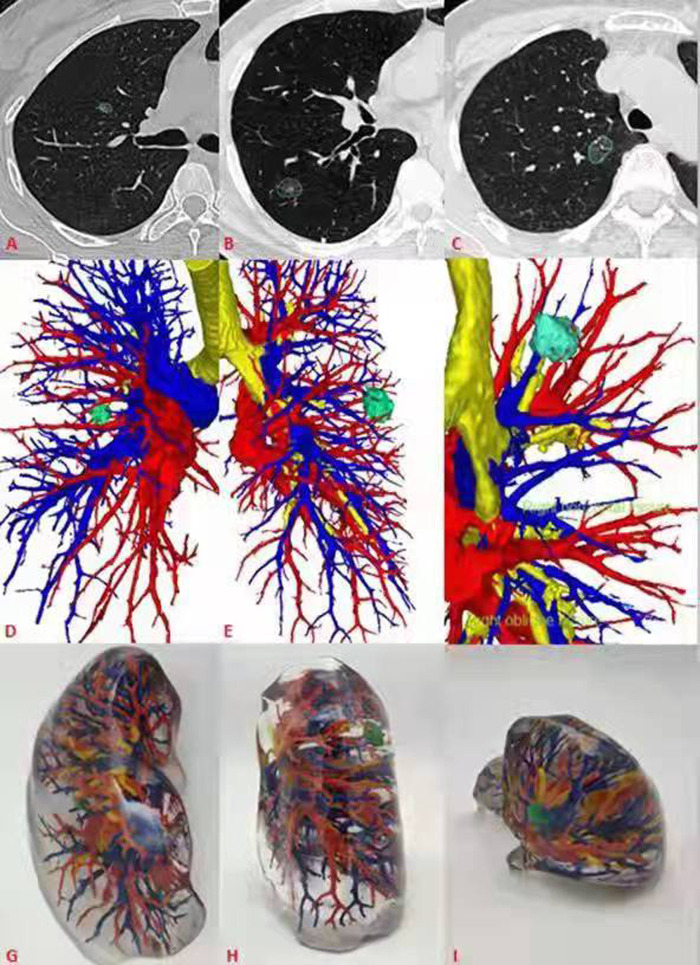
General CT, 3D-CT and 3D printing models of 3 patients. (A–C) General CT showed ground glass nodules in the anterior segment of the right upper lung, back segment of the right lower lung, and posterior segment of the right upper lung respectively. (D–F) 3D-CT showed the relationship between pulmonary nodules and peripheral bronchi and pulmonary vessels. (G–I) The 3D printing model shows the pulmonary nodules and its relationship.
